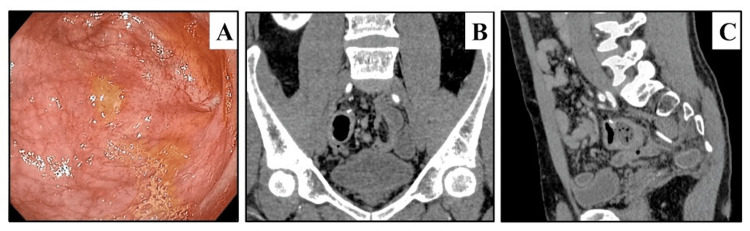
Ten-month follow-up after endoscopic closure Endoscopy showed complete mucosal healing at the ileal pouch stump without recurrent fistula or ulceration (A). Contrast-enhanced CT showed no residual pelvic abscess, free air, or inflammatory changes around the pouch stump (B (coronal view) and C (sagittal view)), confirming durable resolution. CT: computed tomography
